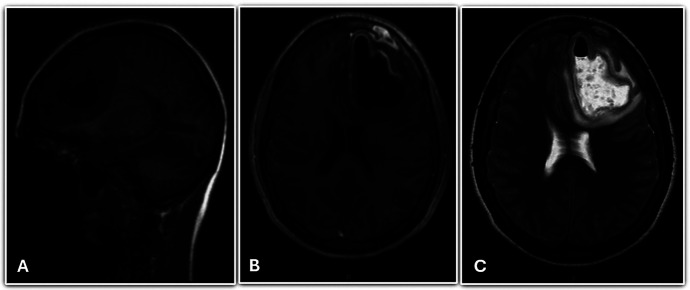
MRI showing A) abscess in the left frontal region; B) expansive formation in the left frontal lobe and frontal sinus discontinuity; and C) hypersignal in the adjacent parenchyma and shift of the midline to the right (contrast).
